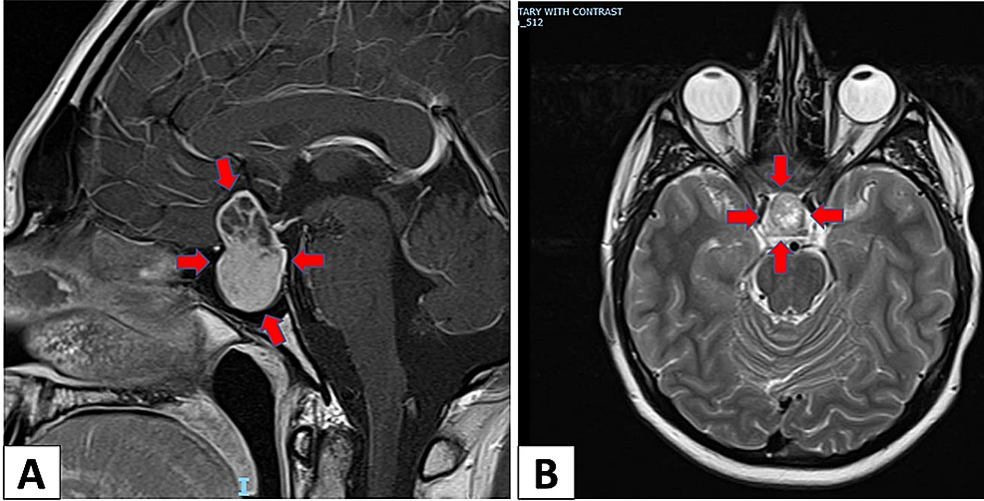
Sagittal (A) and axial (B) sections of brain MRI show 37mm pituitary macroadenoma with suprasellar cystic extension (red arrows)
